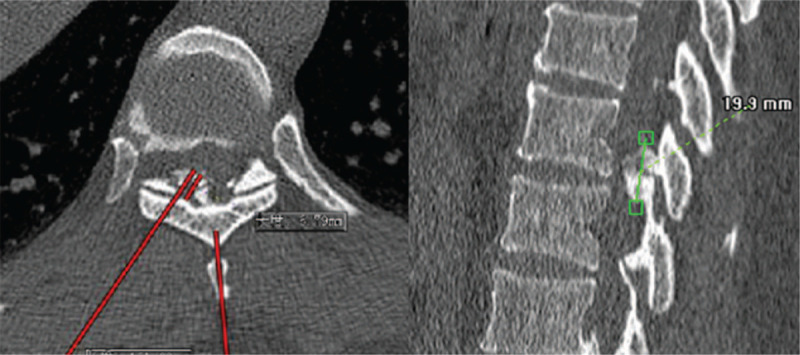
Thickness and height measurement of the ossification on the preoperative CT scans.
